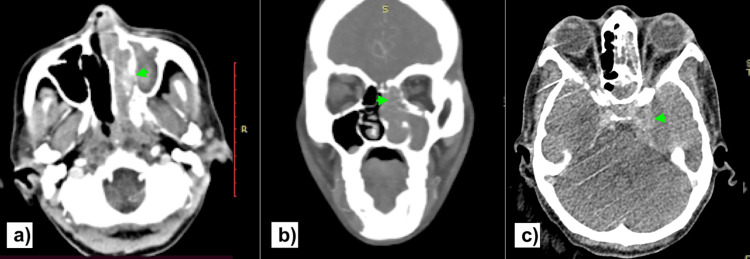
Contrast-enhanced computed tomography of the nose and paranasal sinuses revealed an enhancing soft tissue mass lesion in the nasopharynx extending into the left nasal cavity (a), left maxillary, ethmoid, and frontal sinus (b), and into the middle cranial fossa in the region of the cavernous sinus (c), all marked with green arrowheads.
